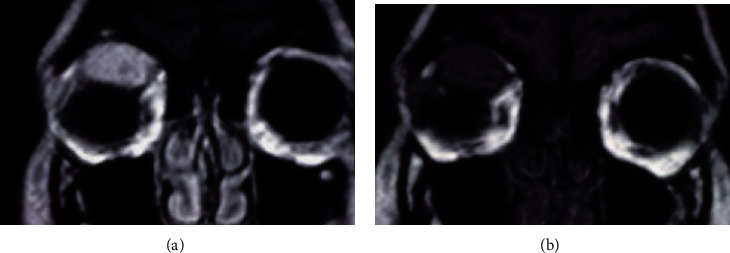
Case 1. (a) Magnetic resonance imaging (MRI) in coronal image showing T2-weighted extraconal tumor based on superior and central orbital, which measures 3.7 cm in the greatest axis and hyperintensity image. (b) T1 weighted with hypointensity image.
